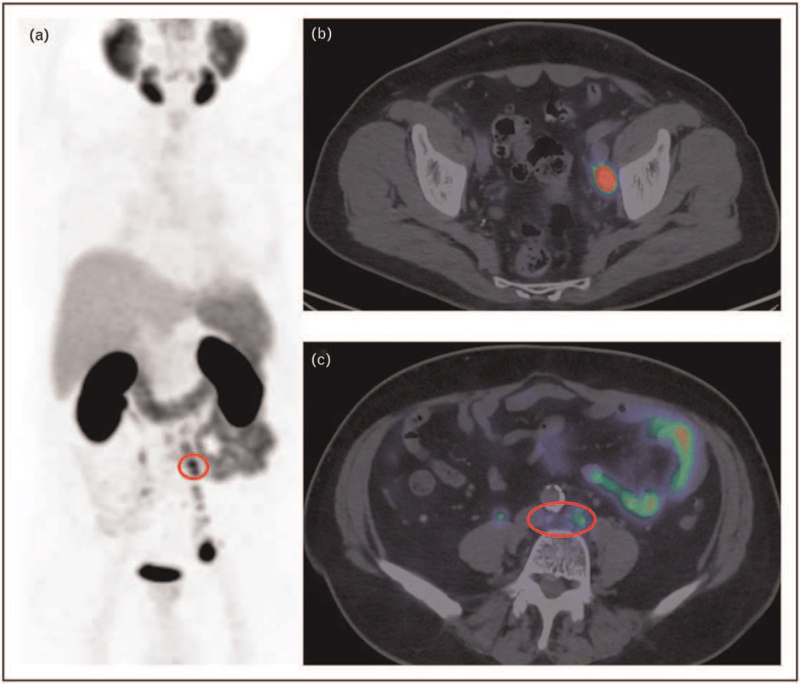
A 75-year-old man with prostate adenocarcinoma (iPSA = 26 ng/ml; GS 4+3). Primary staging ^68^Ga-PSMA PET/CT (a, MIP) showed several lymph node metastasis with increased PSMA uptake in the pelvis (b, fused images with a large left internal iliac node) and in the abdomen (c, fused image, red circle showing some small retroperitoneal nodes with PSMA uptake). Patients was excluded from surgery and subsequentley treated with ADT. ^68^Ga-PSMA PET/CT in primary staging of high-risk patients can easily detect sites of metastasis outside the surgical template, thus excluding those patients with M disease. ADT, androgen deprivation therapy; CT, computed tomography; MIP, maximum intensity projection; PSMA, prostate-specific membrane antigen.
